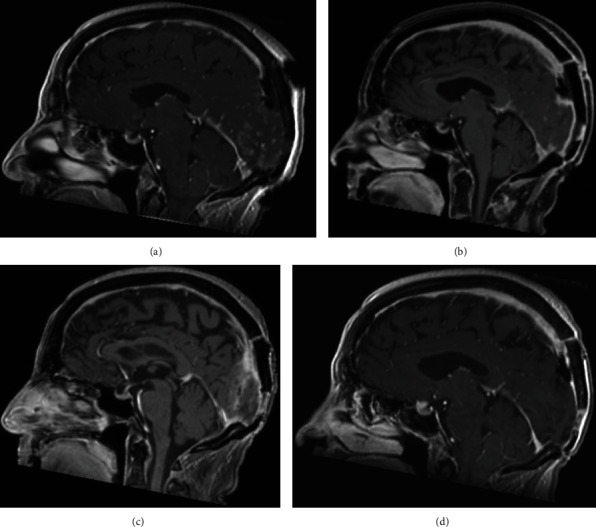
Post-operative MRI confirming the GTR of the tumor with no signs of recurrence at (a) 24 hours, (b) 3 months, (c) 6 months, and (d) 12 months. No signs of cerebral venous infarction after complete removal of the tumor.
